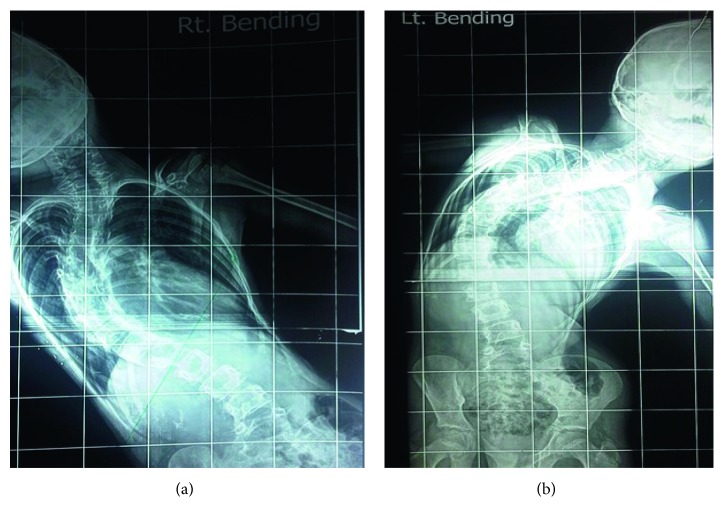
Preoperative X-ray showing right idiopathic thoracolumbar scoliosis.
